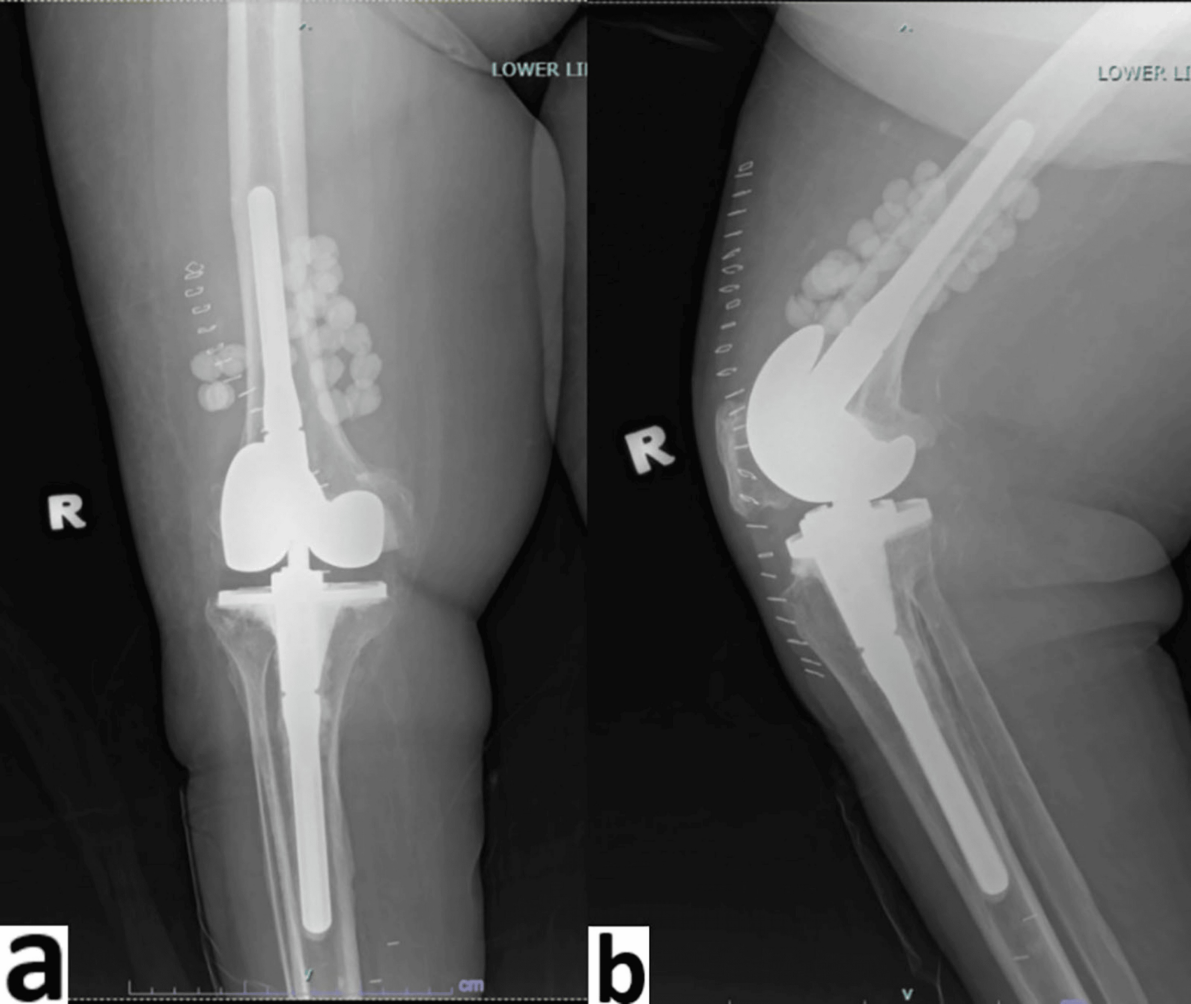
Post-operative radiograph of right knee with vancomycin beads insertion a) anteroposterior view; b) lateral view
